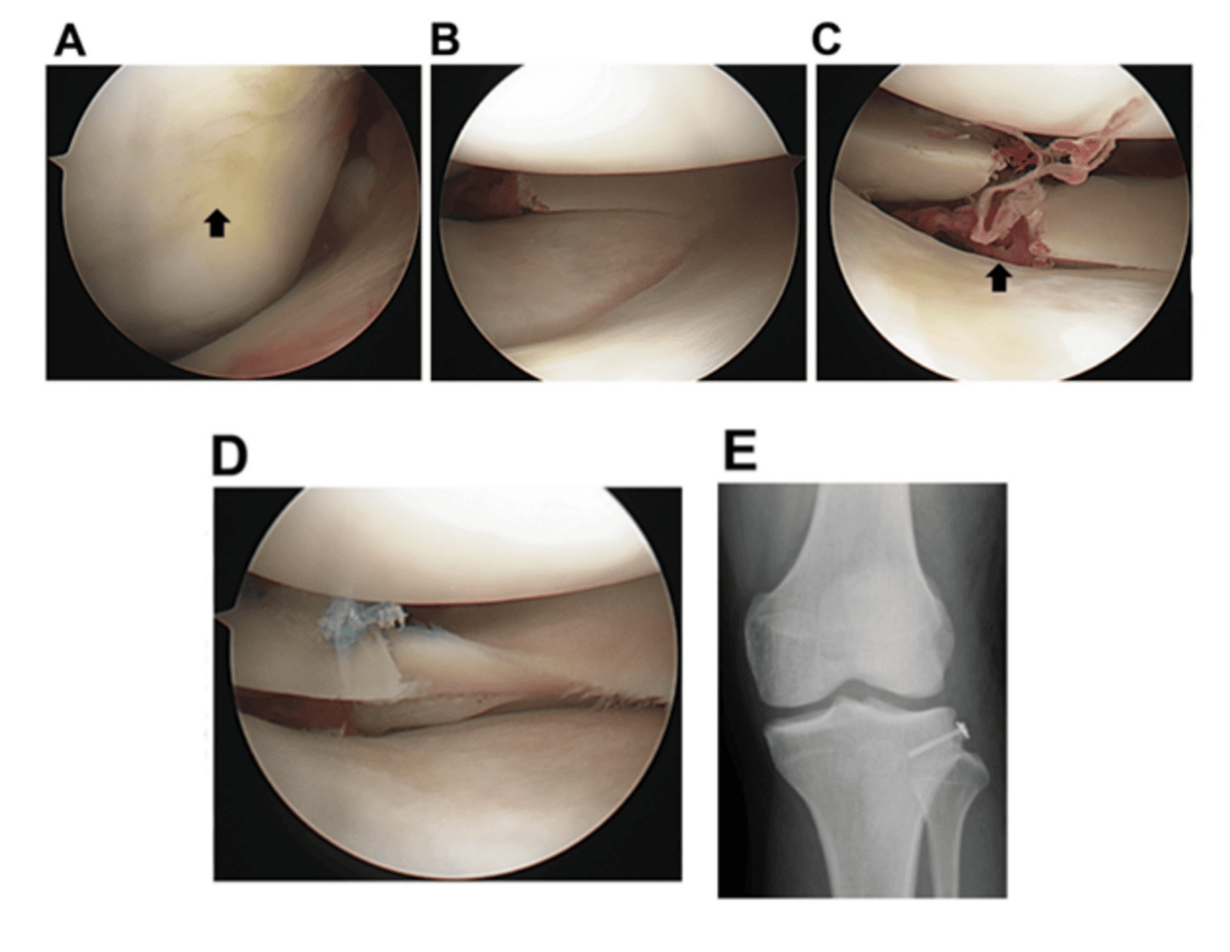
Arthroscopic view images at the time of lateral meniscal repair. (A) ICRS grade I cartilage injury in the weight-bearing area of the femoral side at full extension (black arrow). (B) An arthroscopic view showing no obvious cartilage damage in the tibial side. (C) A complete radial tear of the posterior segment (black arrow). (D) After side-to-side repair of the tear. (E) Anteroposterior view radiograph after the osteosynthesis. ICRS: International Cartilage Repair Society
